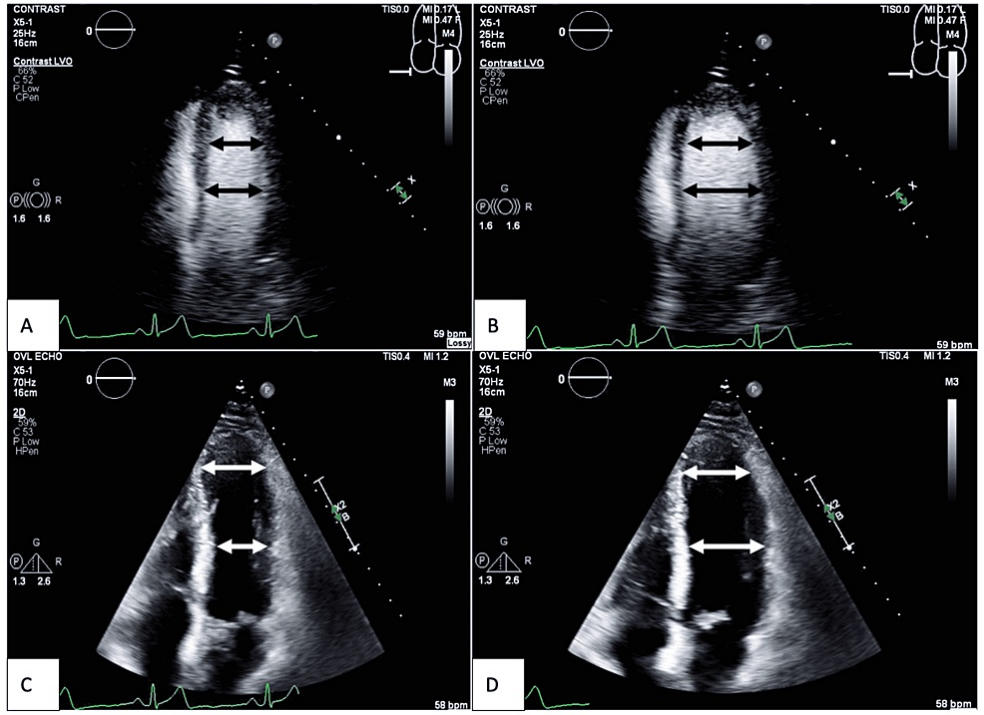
Apical four-chamber view of first follow-up echocardiogram after cardiac catheterization A: Contrast study showing the left ventricle in systole; B: contrast study showing left ventricle in diastole; C: non-contrast study showing the left ventricle in systole; D: non-contrast study showing the left ventricle in diastole. Note that both contrast and non-contrast studies revealed good contraction of the mid-segment of the left ventricle but the diameter (the length of white and black double-headed arrows) of the apical segment in diastole and systole does not significantly change. This demonstrated apical hypokinesis. Ejection fraction had decreased to 40%.
